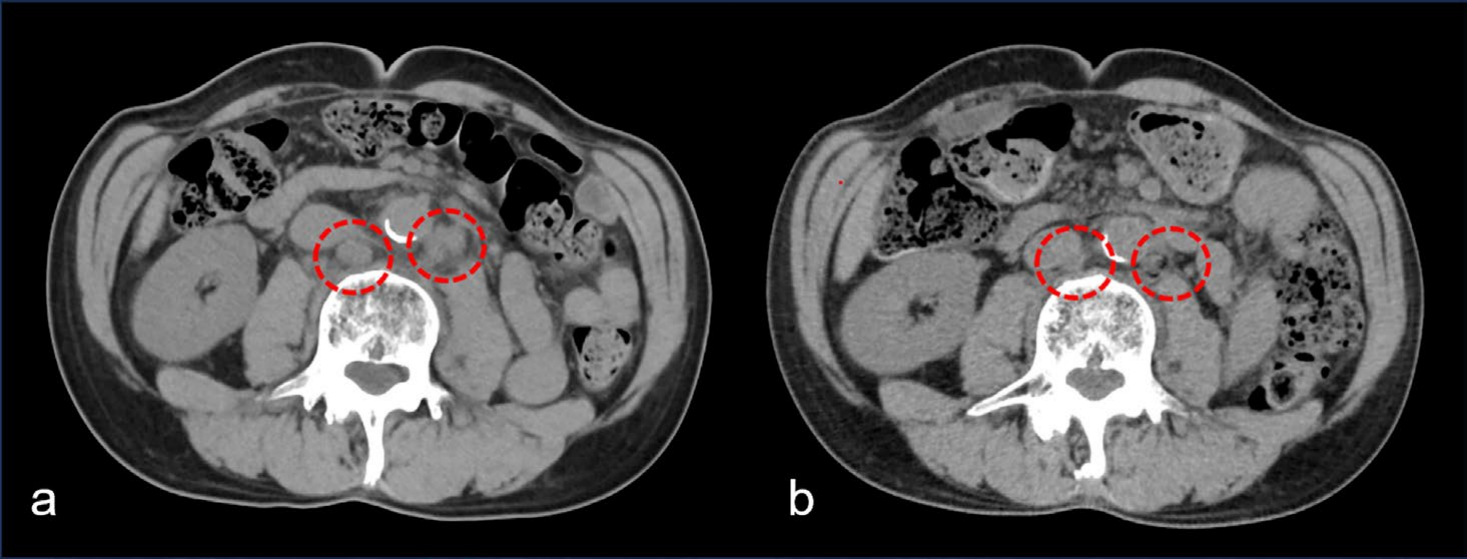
CT images showing recurrent lymphadenopathy and response to systemic therapy. Ten months after surgery, CT scans revealed enlarged para‐aortic and bilateral pelvic lymph nodes (a). Following three cycles of enfortumab vedotin plus pembrolizumab, the metastatic lymph nodes showed marked reduction in size consistent with a complete response to systemic therapy (b).
